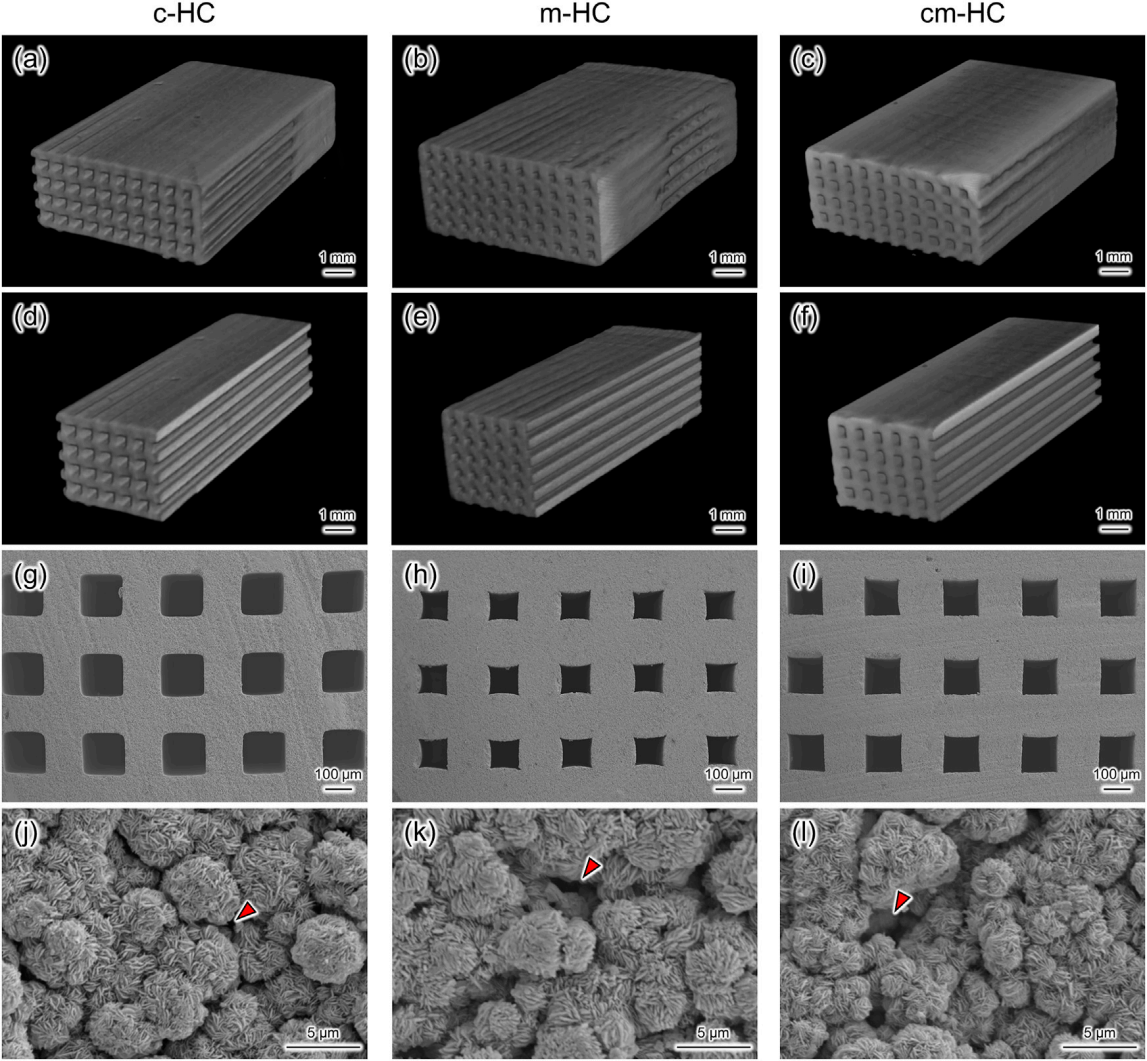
Three-dimensional µ-CT images of (A) c-HC, (B) m-HC, and (C) cm-HC. (D–F) Cross-sectional images of (A–C), respectively. SEM images in the channel aperture region of (G) c-HC, (H) m-HC, and (I) cm-HC at low magnification. SEM images in the struts region of (J) c-HC, (K) m-HC, and (L) cm-HC at high magnification. Red arrowheads show the micropores formed in the struts of CAp HC scaffolds.
